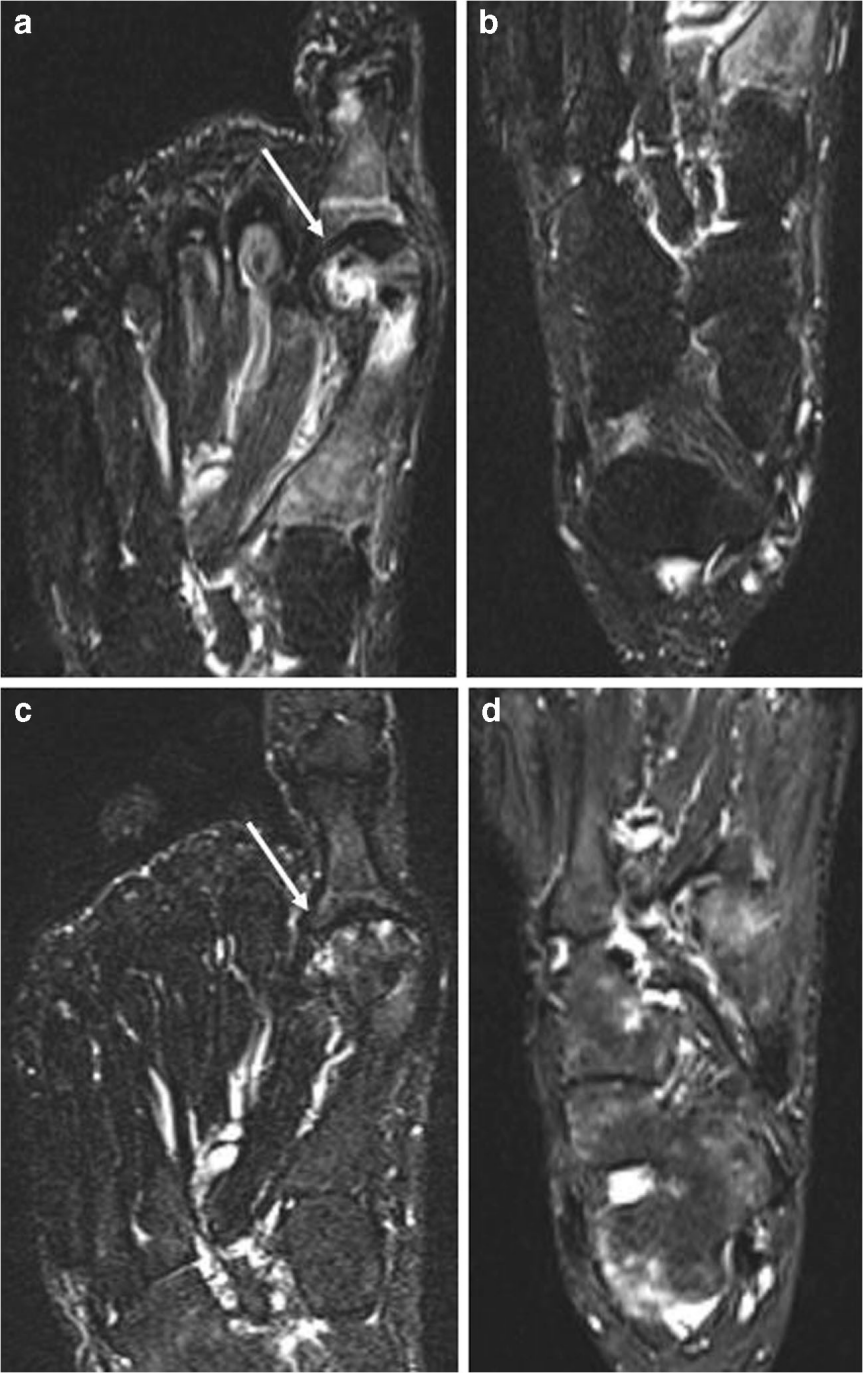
a, b, c, d Traumatic (CRPS) and iatrogenic. A 58-year-old woman that underwent surgery for left hallux valgus correction. All the figures are STIR images. Panels a and b come from the first exam, few weeks after surgery, where it is possible to observe an ELMSI involving the first metatarsal head (*arrow* in a) compatible with the post-surgery (iatrogenic) ELMSI; no ELMSI of the tarsal bones (b). After 6 months, the patient continued to feel pain in the left foot and MRI was repeated. MRI shows the almost total remission of the ELM in the surgical site (*arrow* in c), confirming the alteration was a simple post-surgery (iatrogenic) ELMSI, but its presence with “patchy” distribution in the cuneiform bones, in the cuboid, in the talus, in the calcaneus, associated with effusion in the tarsal and ankle joints (d), led to the diagnosis of CRPS
